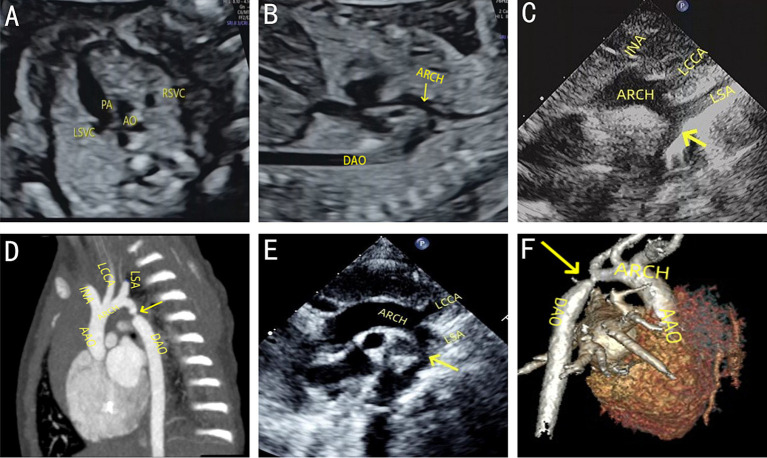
CoA prenatal ultrasound and postnatal imaging. (A) The pulmonary artery is larger than the aorta, and the superior vena cava are double. (B) The aortic arch is tortuous, rigid, and narrow. (C) Preoperative ultrasound showed narrowing of the descending aorta. (D) Preoperative CTA showed narrowing of the descending aorta. (E) Postoperative ultrasound showed residual obstruction in the descending aorta. (F) Postoperative follow-up CT three-dimensional reconstruction of residual obstruction in the descending aorta.
